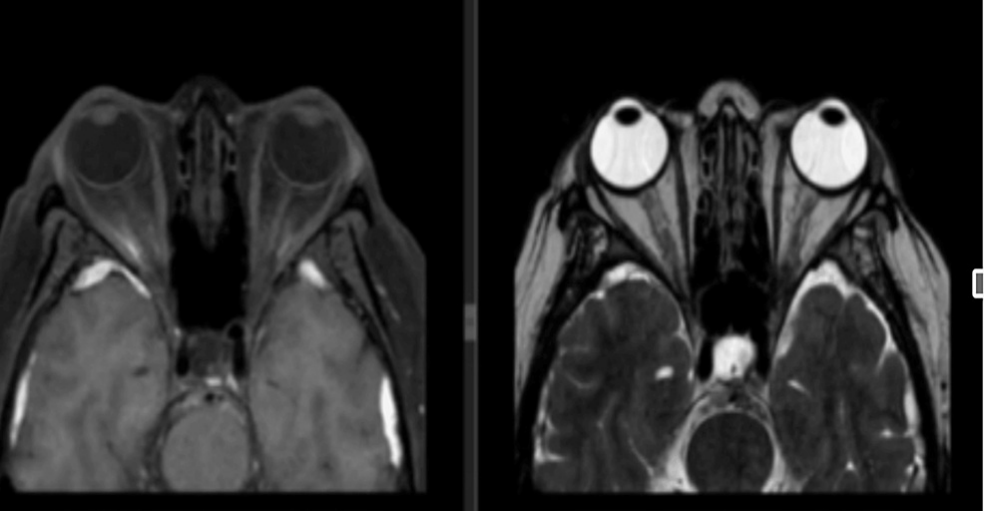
Orbital MRI revealing no distinct mass or enhancing lesions, normal extraconal, intraconal, preseptal, and inferior or infraorbital regions; symmetrical extraocular muscles; and normal-sized optic nerves. Right: T2-weighted. Left: FLAIR: fluid-attenuated inversion recovery.
